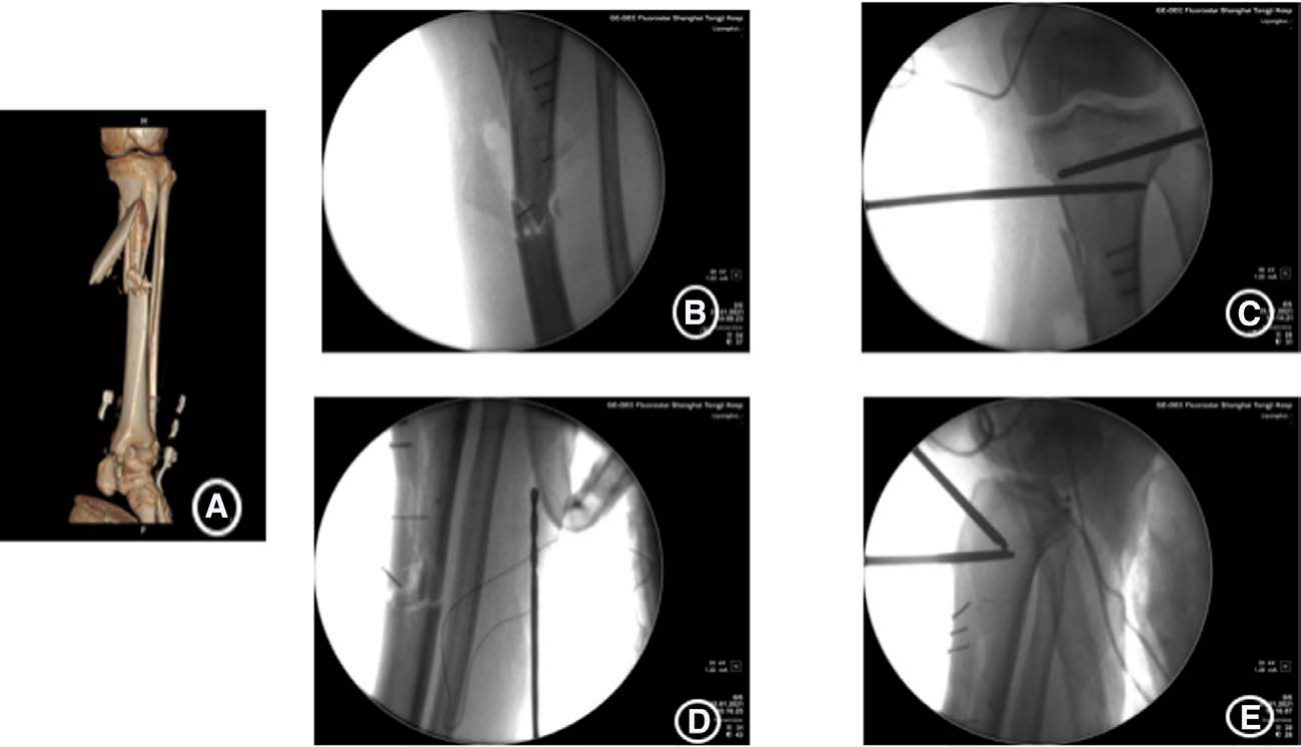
(A) 3-D CT of the fracture. (B–E) C-arm image of the fracture reduction. CT = computerized tomography.
